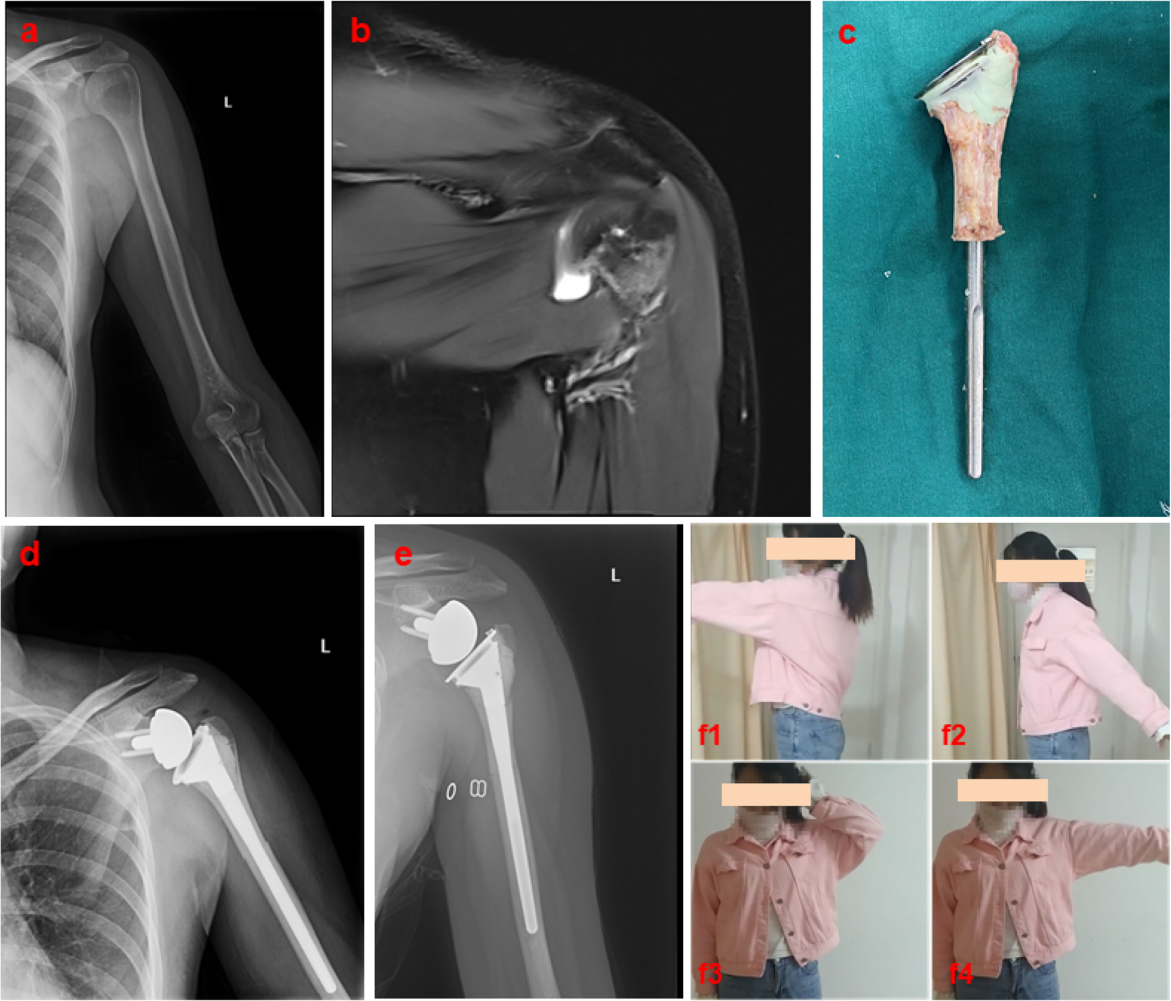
Case 2 (a, b) Preoperative AP X-ray and MRI indicate an osteogenic lesion and the pathological fracture in the left caput humeri. (c) Surgically inactivated isolated autogenous graft. (d) Postoperative AP X-ray. (e, f1-4) Six months later, the postoperative X-ray image and functional follow-up indicated stable joint function and alignment
